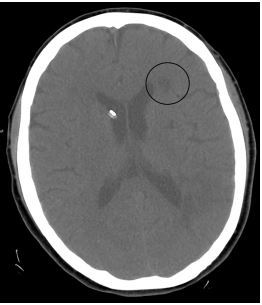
Non-contrast computed tomography brain demonstrating a low attenuated area of the left frontal lobe in a middle cerebral artery watershed area (circle).
